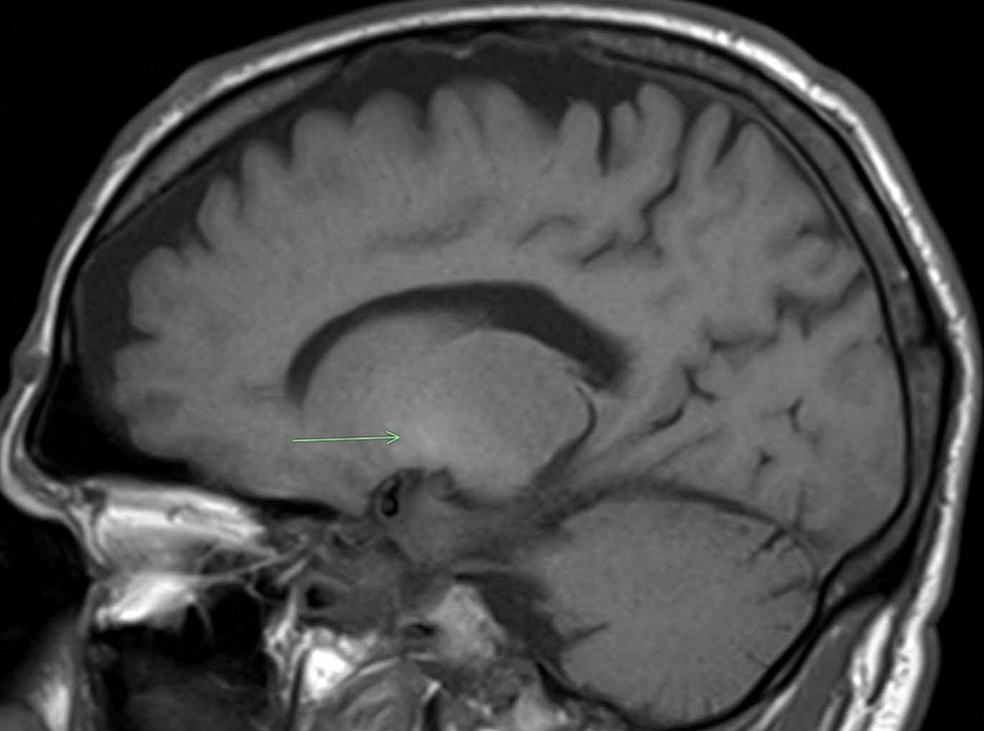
MRI brain in the left sagittal view demonstrating subtle pre-contrast T1 hyperintensity in the left basal ganglia (green arrow).
